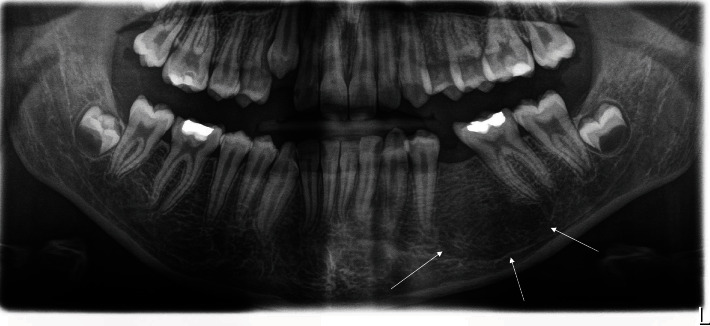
Control OPG X-ray showing good bone healing, with no signs of relapse.
